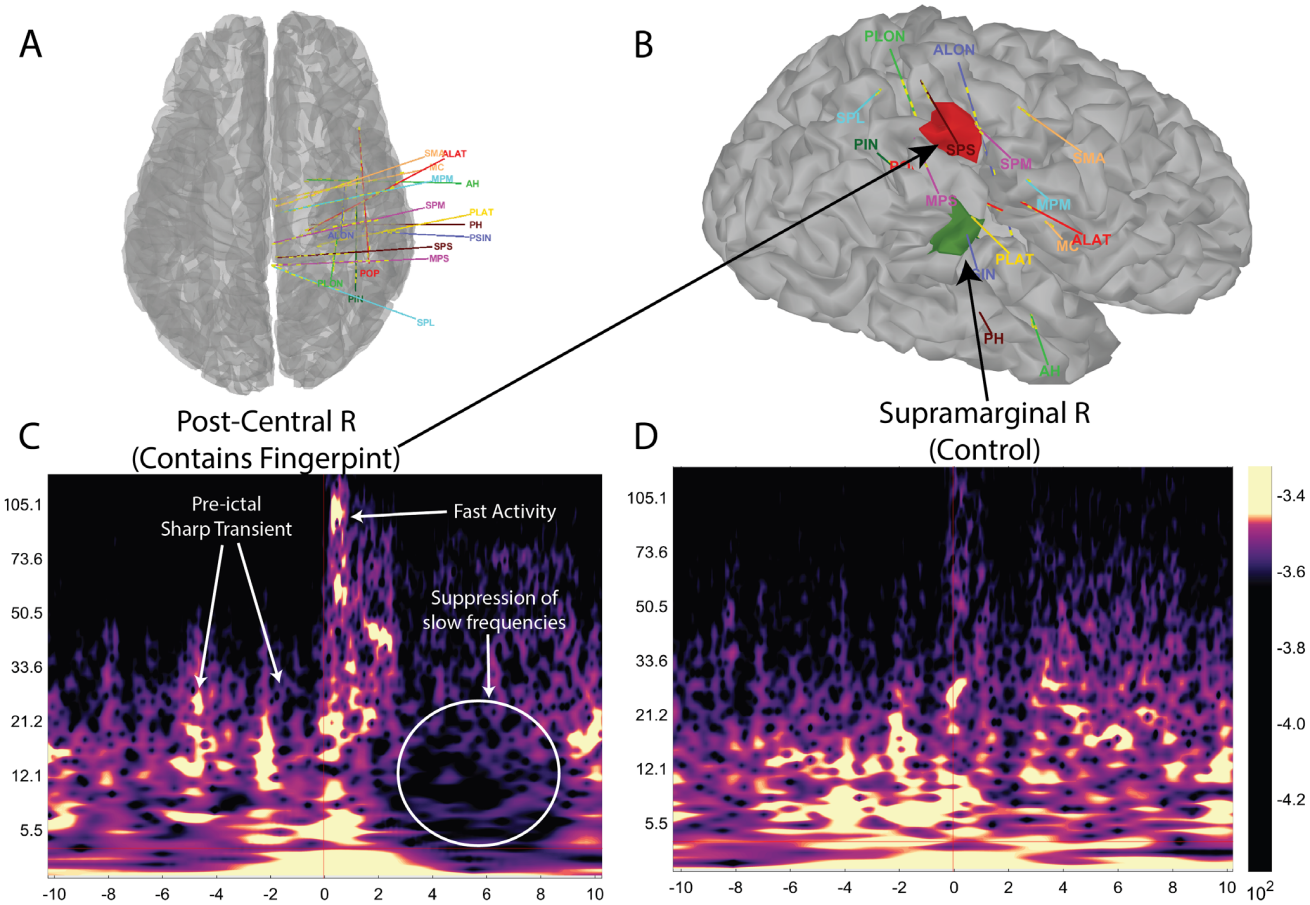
(A) Superior view and (B) Lateral view of cortex with extracted from preimplant MRI showing the SEEG implants. (C) Postcentral R region showing the seizure fingerprint. (D) Supramarginal R region acting as a control.
